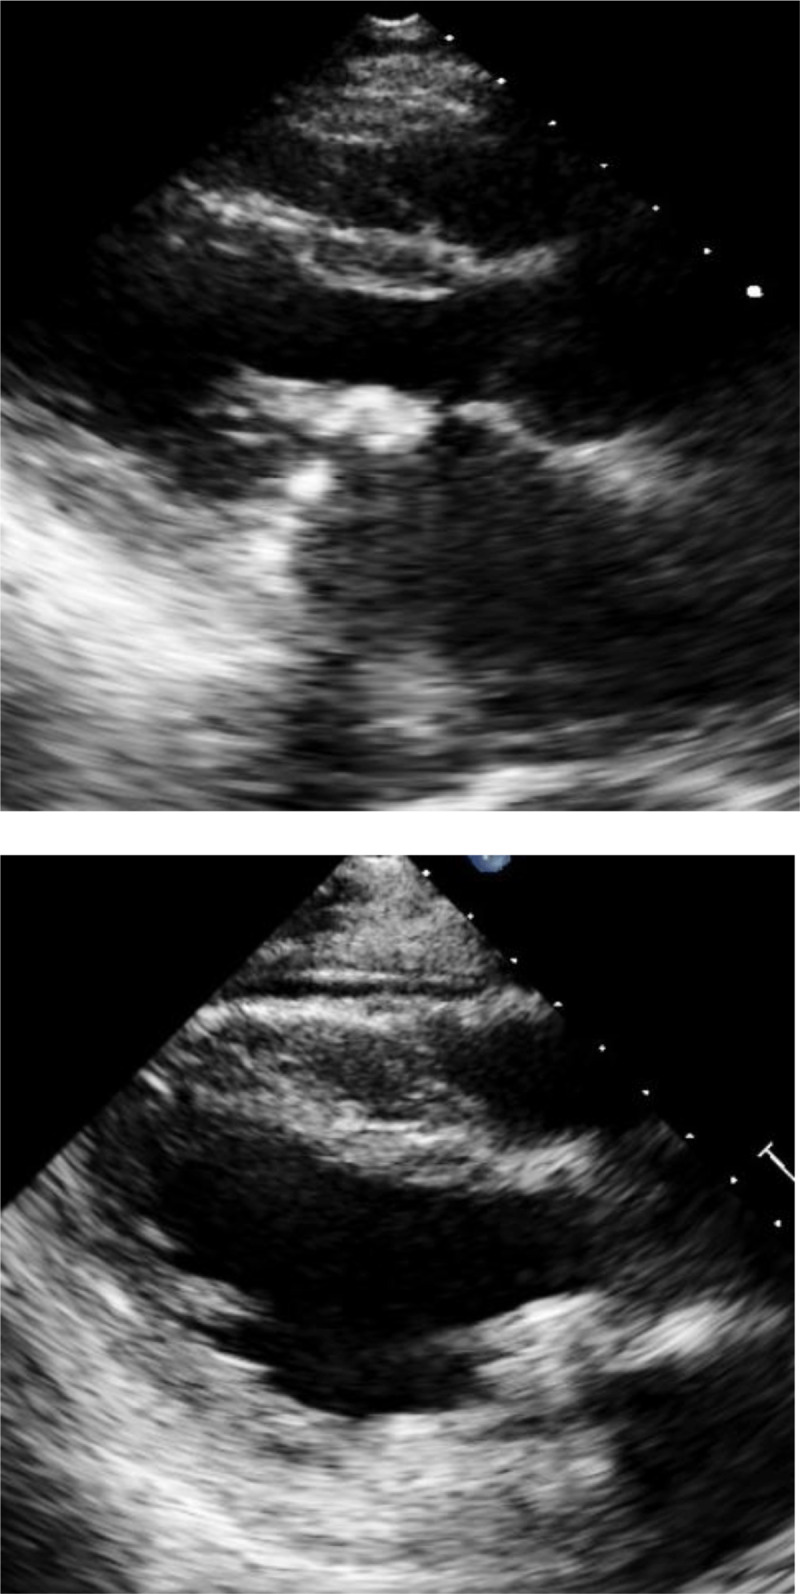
The echocardiographic pictures of rheumatic mitral valve disease pre (above picture) and post replacement with mechanical prosthetic valve (below picture).
